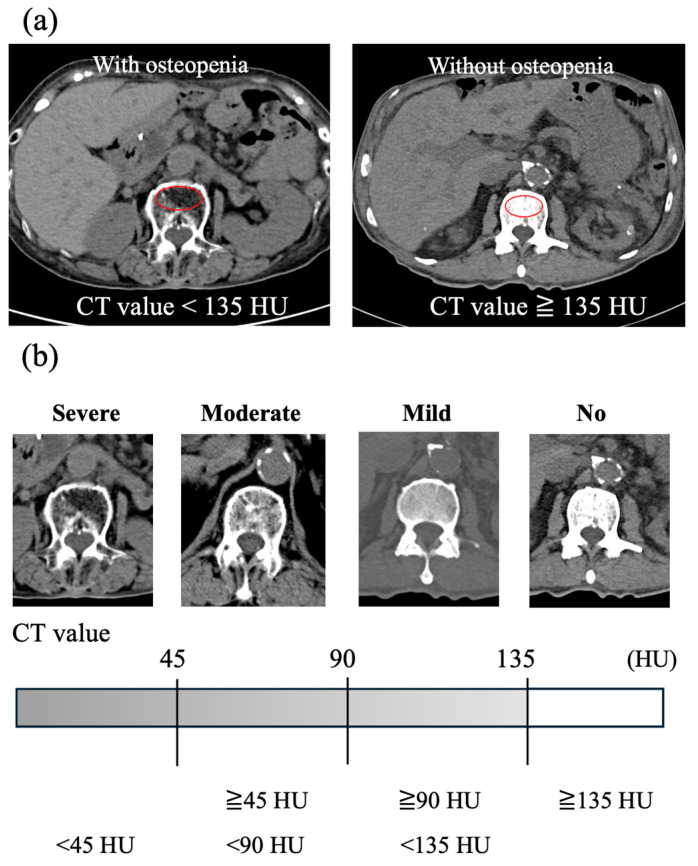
Trabecular L1 CT attenuation value for bone mineral density assessment using CT scan images. (a) Examples of axial CT images at the L1 vertebral level in patients with and without osteopenia. The red circle indicates the region of interest for measuring the average CT attenuation of the vertebral trabecular bone. (b) Severity of low bone mineral density according to the average CT value of the vertebral trabecular bone. CT, computed tomography; HU, Hounsfield units.
